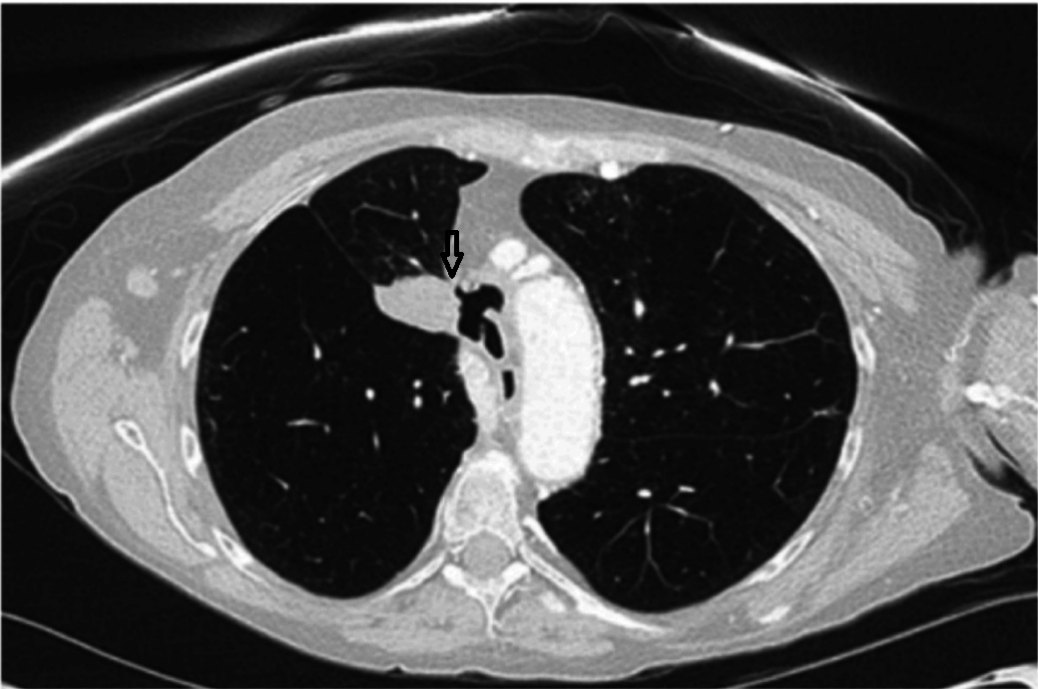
Computed tomography (CT) scan showing tumor near the mediastinum and formation of a fistula.
